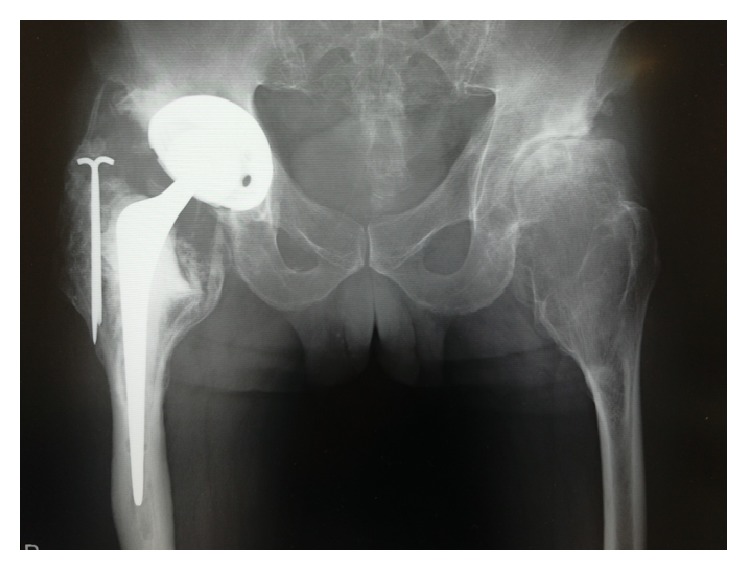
Anteroposterior radiograph of the pelvis taken 13 months postoperatively showing ectopic ossification.
